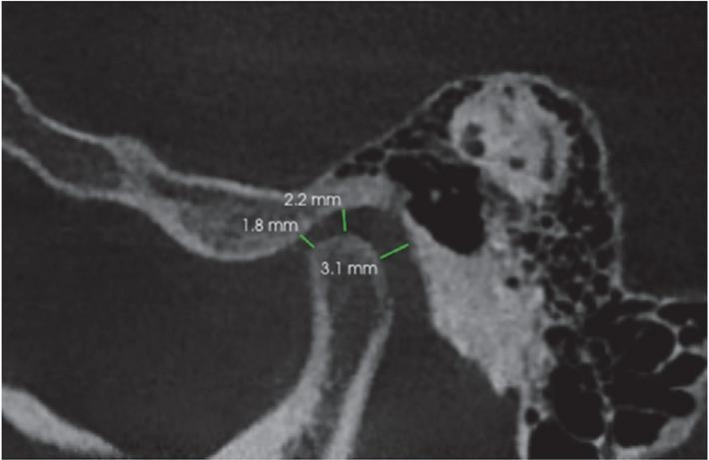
Sagittal section of TMJ in CBCT showing joint space dimensions.
